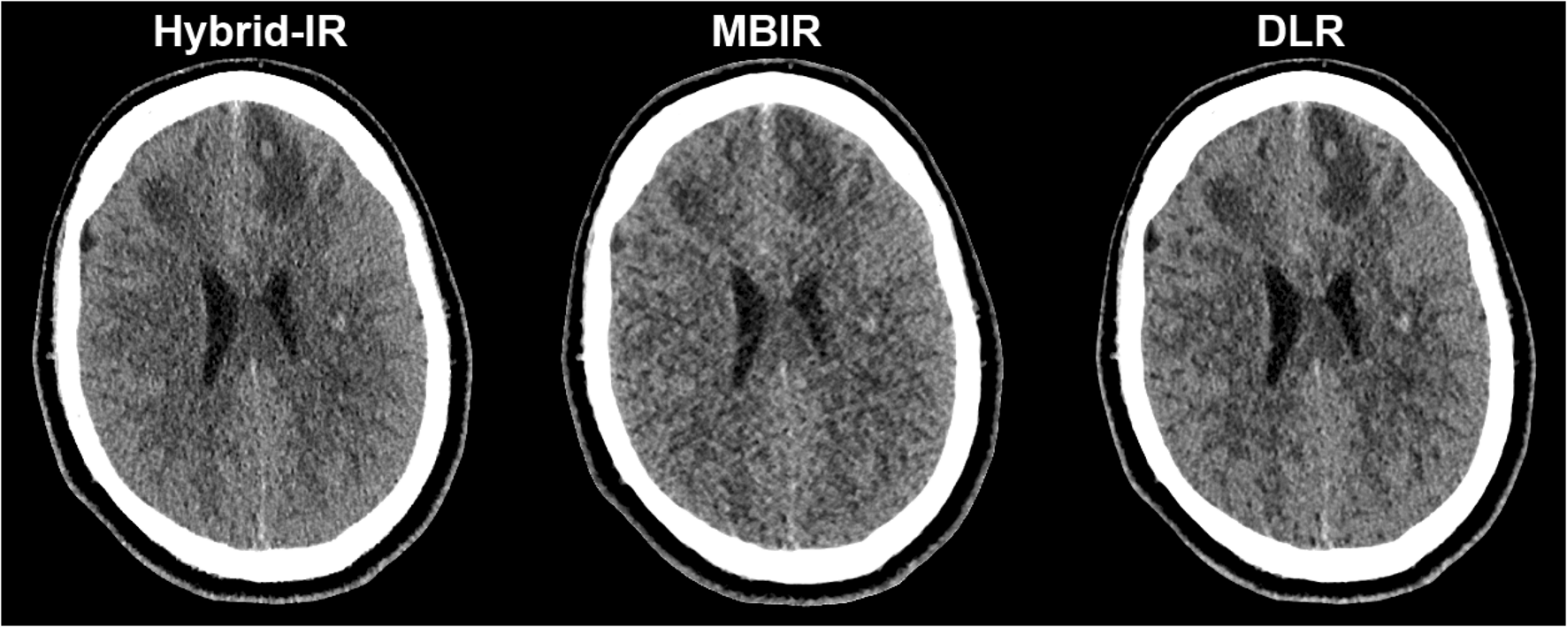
Example slice demonstrating the impact of the different reconstruction algorithms for the same non-contrast CT acquisition in a patient with small intracerebral haemorrhages with surrounding oedema
